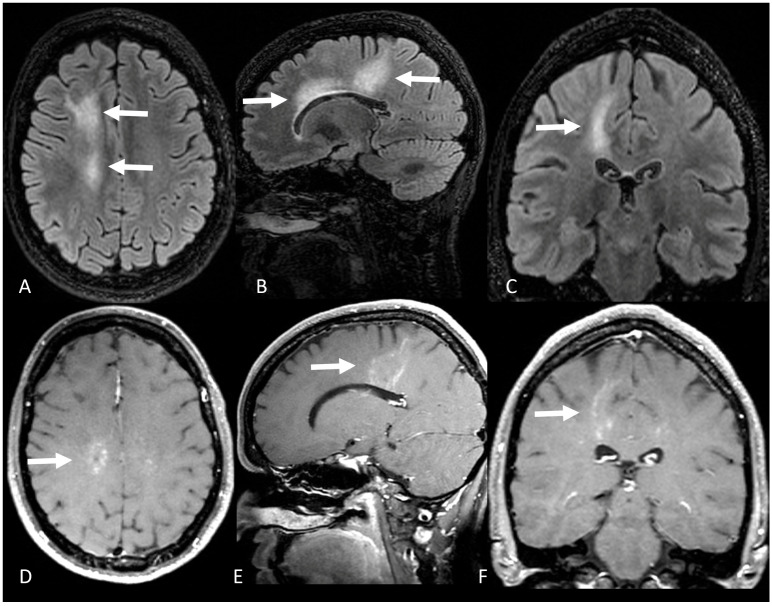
(A) Axial, (B) sagittal, and (C) coronal FLAIR shows hyperintensity in the right fronto-parietal subcortical and periventricular white matter (white arrows). (D) Axial, (E) sagittal, and (F) coronal T1-weighted gadolinium-enhanced images shows patchy nodular and curvilinear pattern of contrast enhancement in the right fronto-parietal subcortical and periventricular white matter (white arrows).
